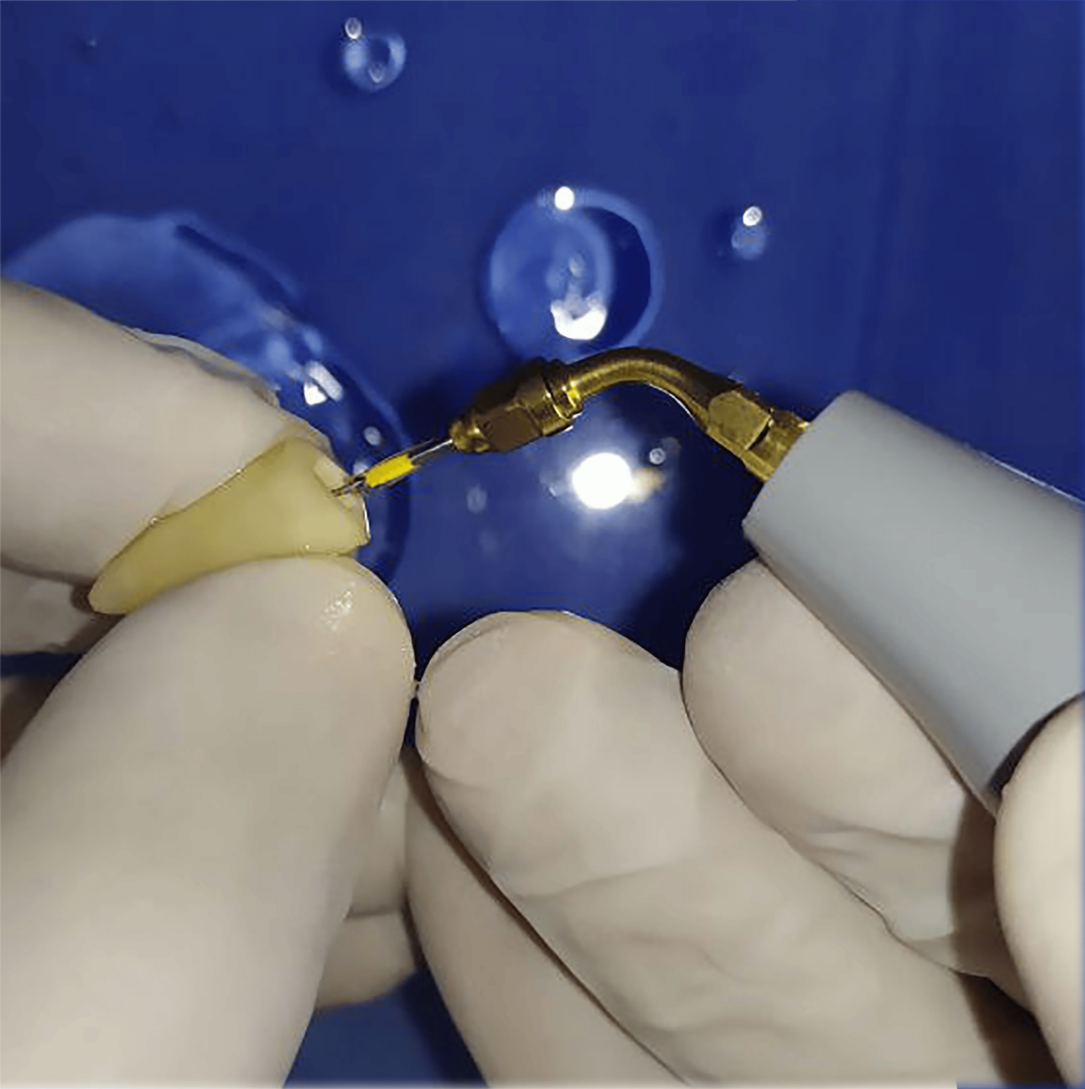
Ultrasonic activation during the root canal preparation.
